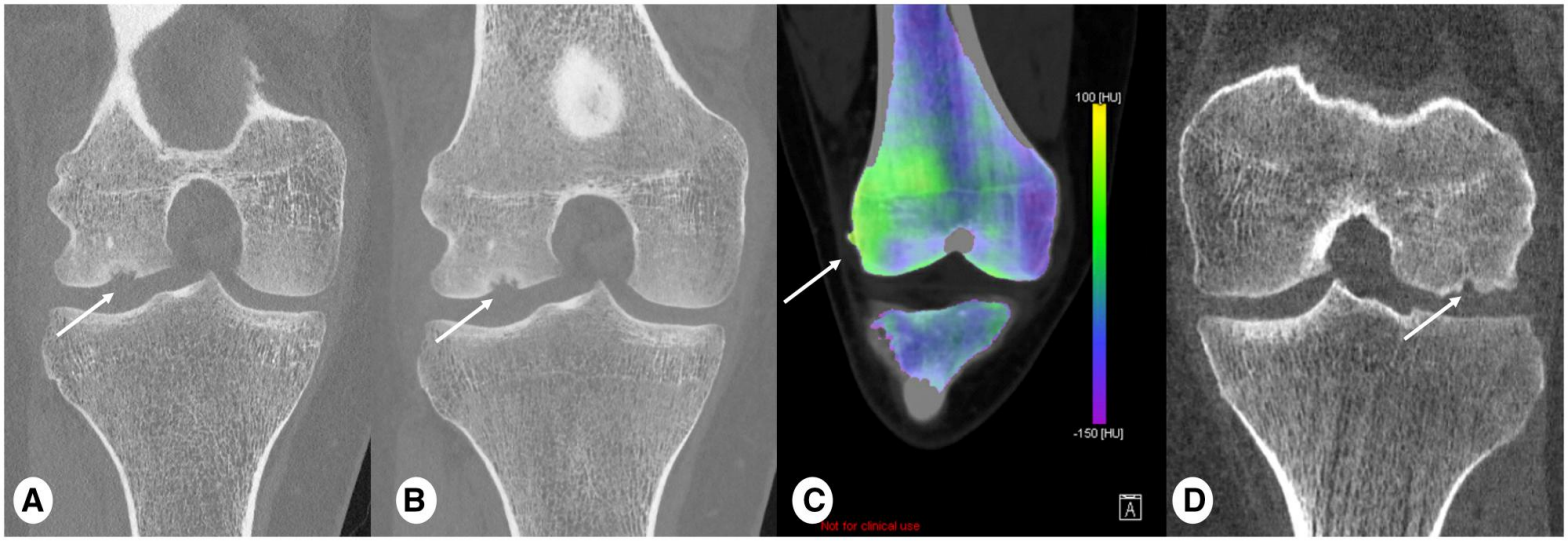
Comparison PCD-CT and EID-CT to assess osteochondritis dissecans (OCD). (A) 0.2 mm and (B) 0.6 mm coronal PCD-CT images (reconstruction kernel Br89 and IR level 3, Q3) and (C) Virtual Non-Calcium (VNCa) imaging for bone marrow oedema detection 3 mm multiplanar reformat in a 16 years old male showing cortical defect at the lateral femoral condyle of the right knee (arrows in A and B) after sport trauma. (D) Coronal EID-CT (slice thickness 0.6 mm, reconstruction kernel Br89 and IR 3, Q3) in a 16 years old male showing a cortical defect at lateral femoral condyle of the left knee (arrow). Note clear definition of the cortical contours of OCD lesion on image (A) and (B) and reduced noise at the same slice thickness ((B) vs. (D)). Interestingly, total dose-length product (DLP) was 200 mGy×cm and 522 mGy×cm for PCD-CT and EID-CT, respectively. Colour maps in (B) is showing higher density of lateral femoral condyle of the right knee (green colour/arrow) due to higher water content for bone marrow oedema. VNCa reconstruction for bone marrow oedema are still provided as non-clinical/research tool on Naeotom Alpha scanner (Siemens Healthineers, Erlangen, Germany).
